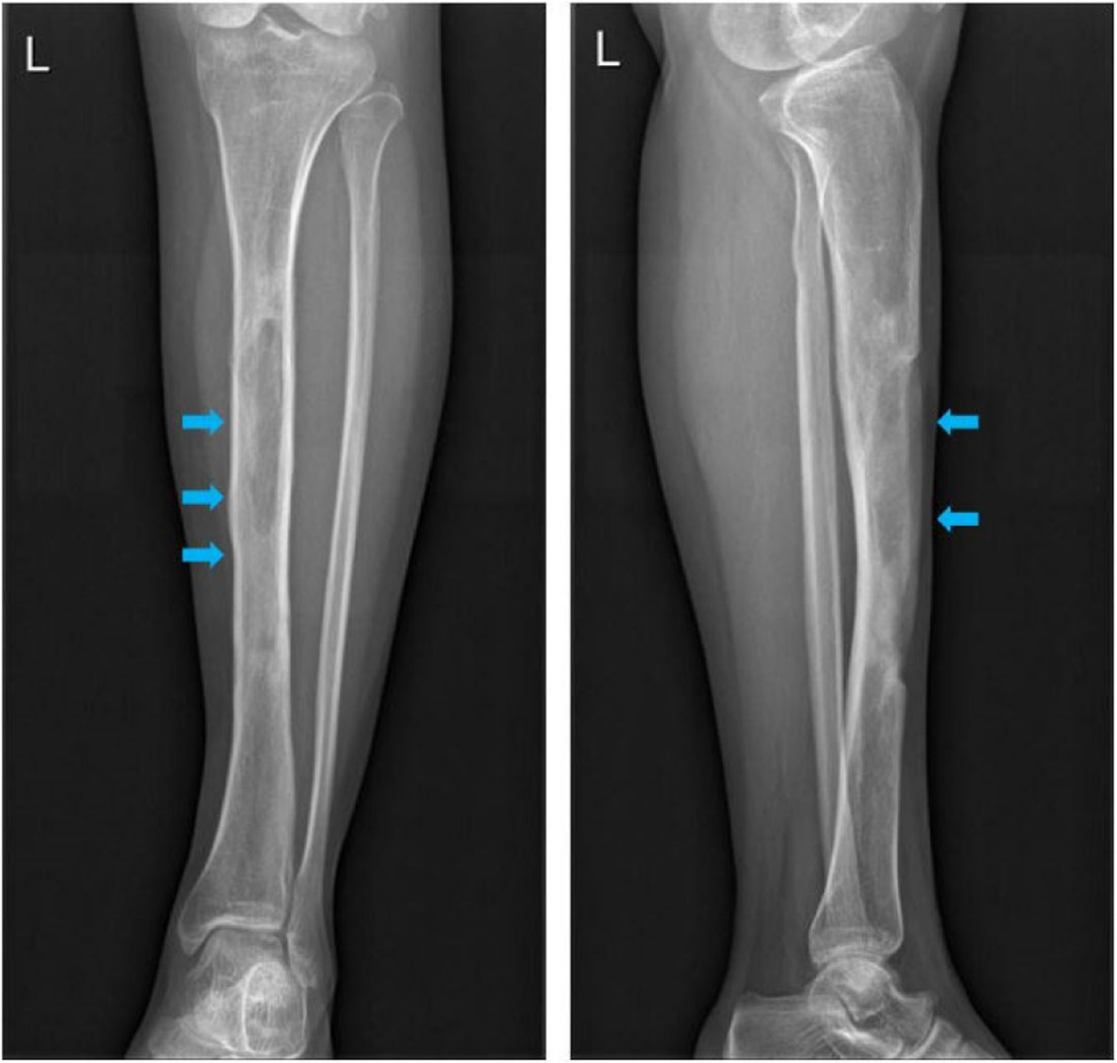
A patient received local debridement and calcium sulfate implantation. The X-ray after 6 months showed that the shape of tibia shaft was not well-restored to the initial, but no fracture was detected
